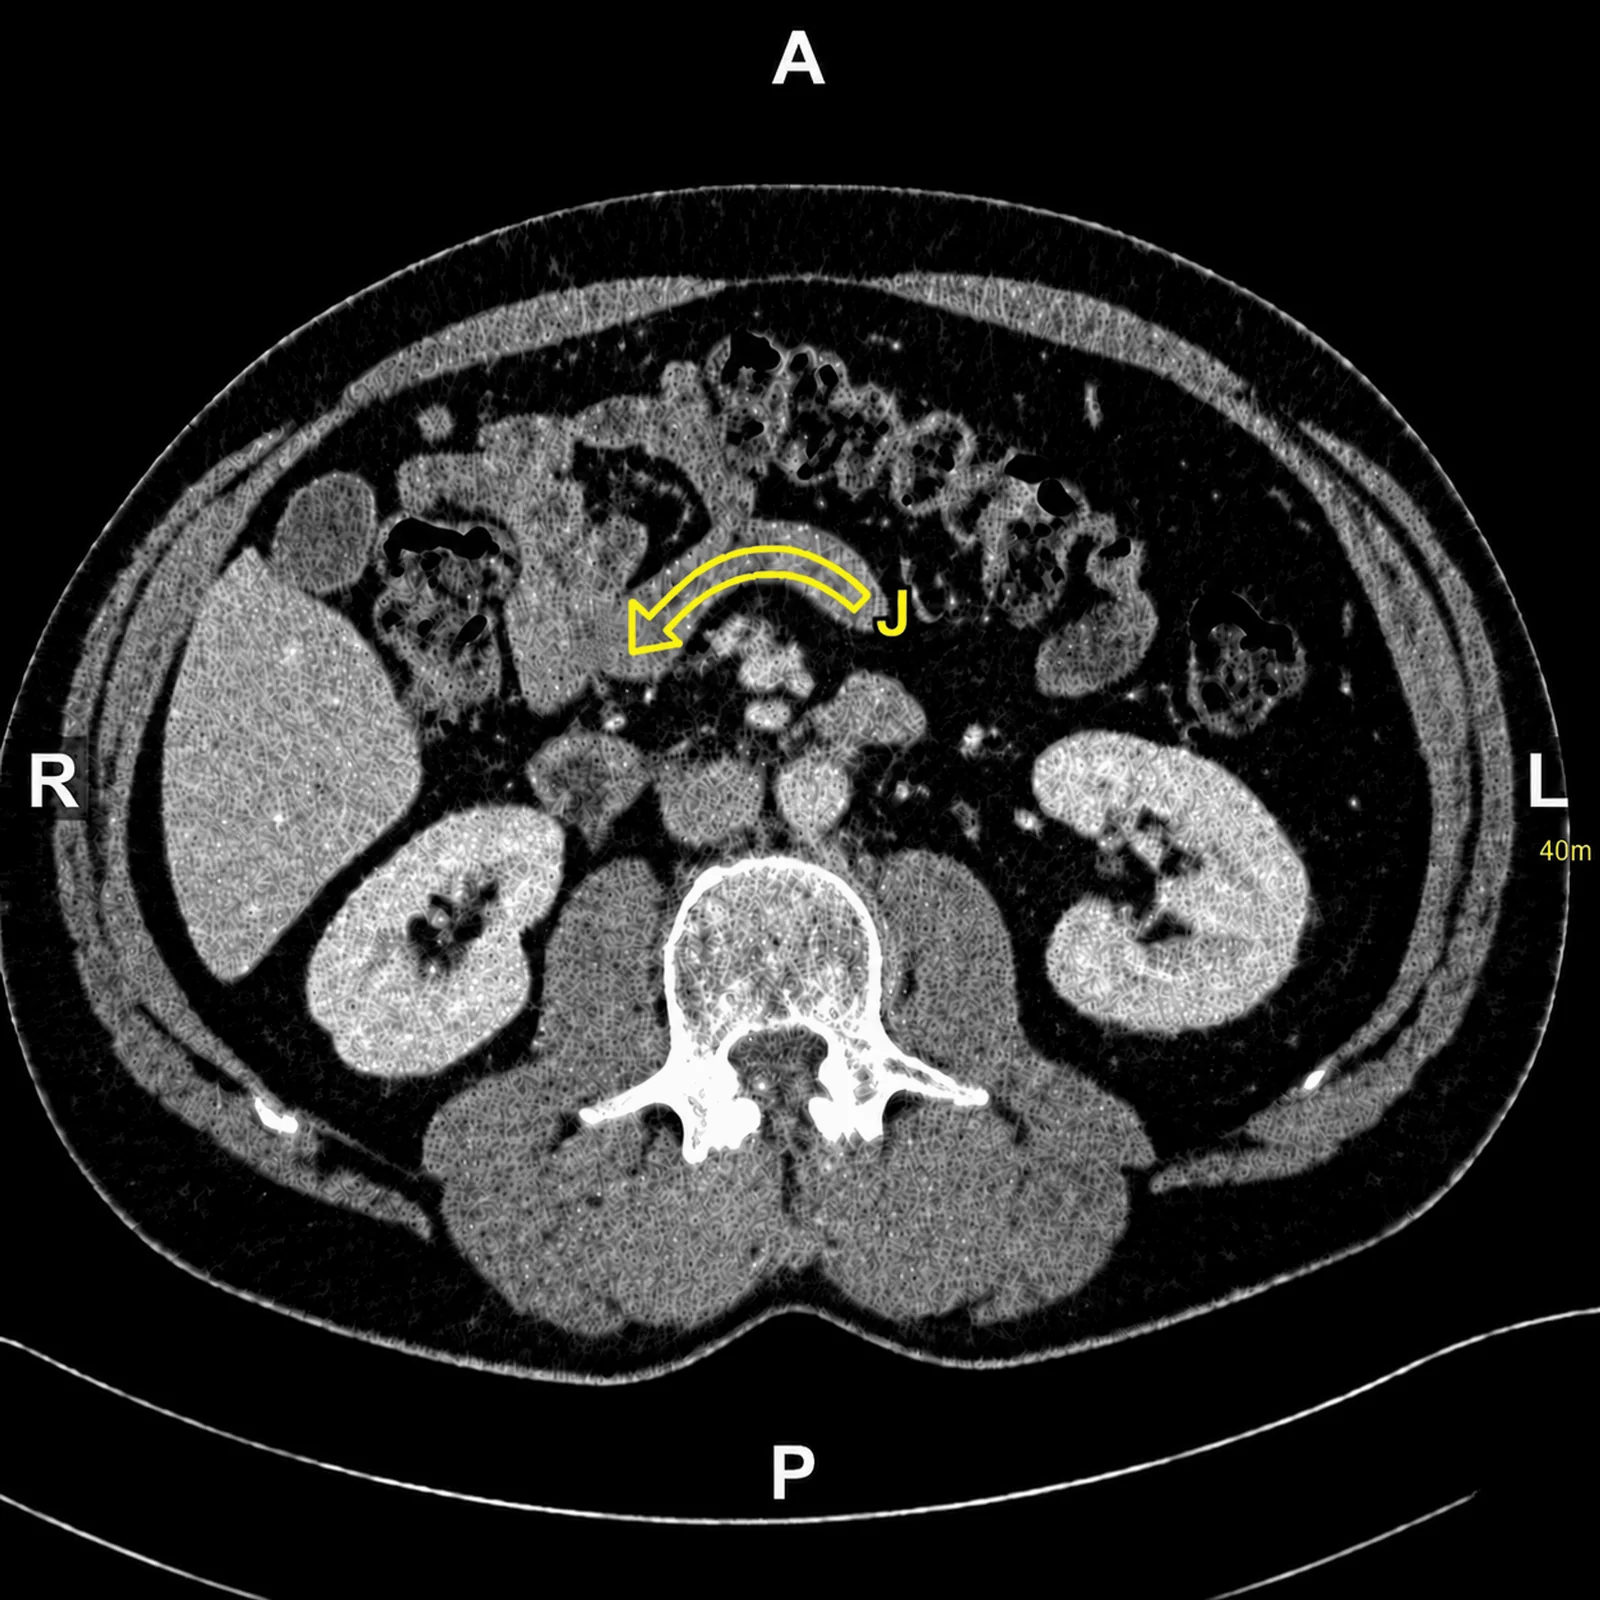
Axial contrast-enhanced CT image of patient 2. The first jejunal loop courses anterior to the superior mesenteric vessels toward the right side, where the remaining small-bowel loops included in the examination are also located, representing features consistent with intestinal malrotation. The colon within the examined region is in a typical anatomical position. The yellow arrow indicates the lumen of the first jejunal loop, which is directed toward the right side. The yellow curved arrow labeled J indicates the lumen and rightward course of the first jejunal loop. R, L, A, and P indicate the patient’s right, left, anterior, and posterior sides, respectively. CT: computed tomography
